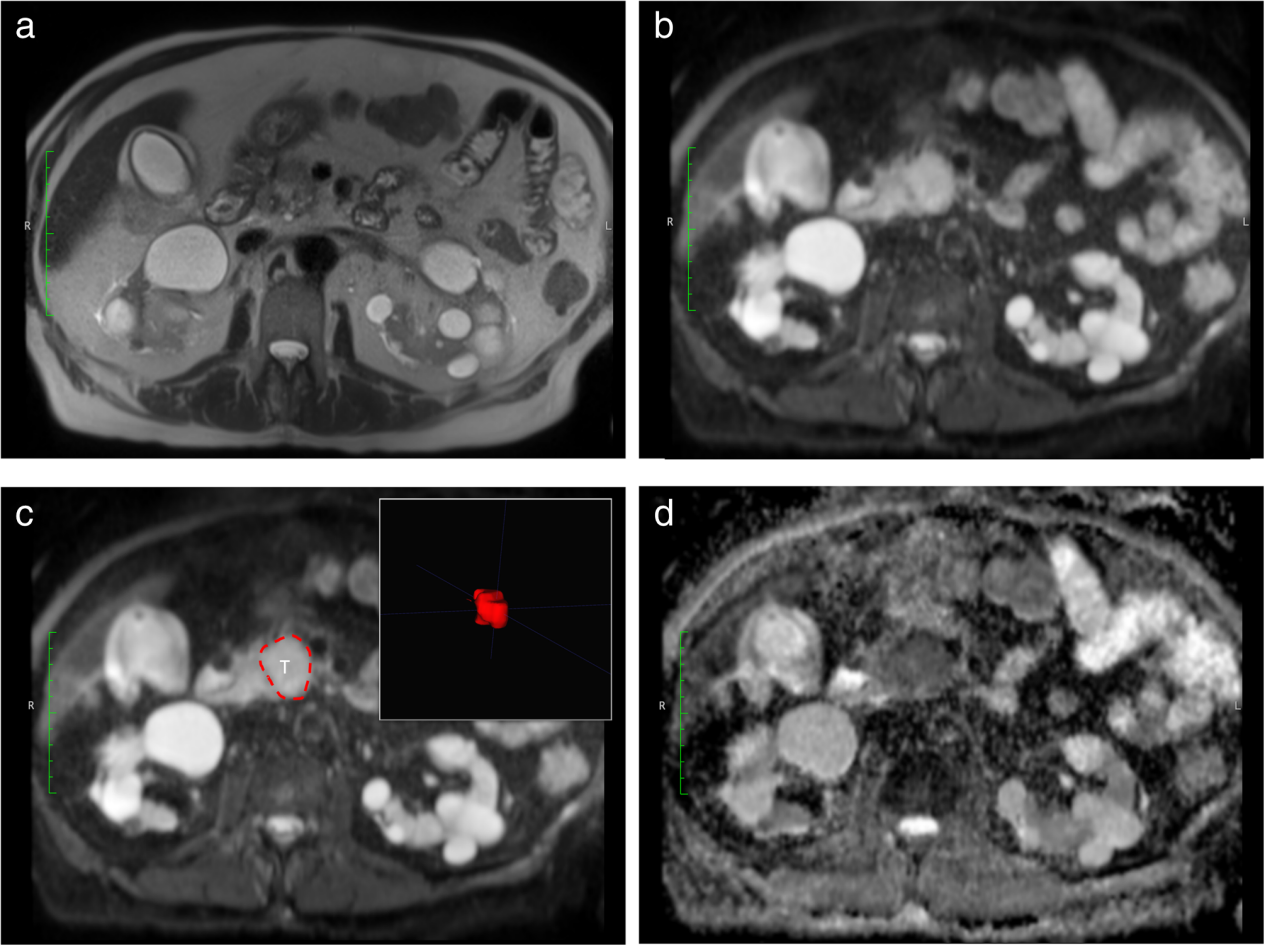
Exemplary case showing a ductal adenocarcinoma of the pancreatic head on T2-weighted images (a), *b* = 600 s/mm^2^ (b), the segmentation image including a three-dimensional rendering (inset) and a region-of interest (T) of the tumor (c), and the apparent diffusion coefficient map (d)
